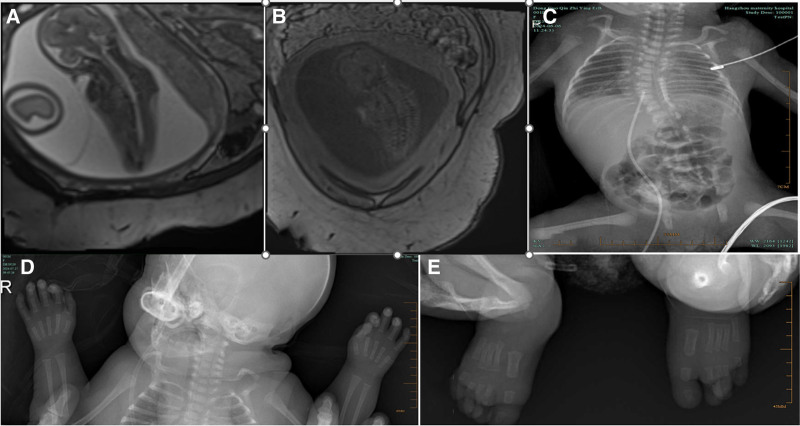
(A and B) MRI revealed spinal scoliosis, thoracolumbar kyphosis, hypoplastic thoracic vertebrae, and vertebral fusion. (C) Chest radiography confirmed scoliosis. (D and E) Wrist and ankle radiographs showed distal flexion contractures of the fingers. No evidence of carpal or tarsal bone fusion was observed. MRI = magnetic resonance imaging.
